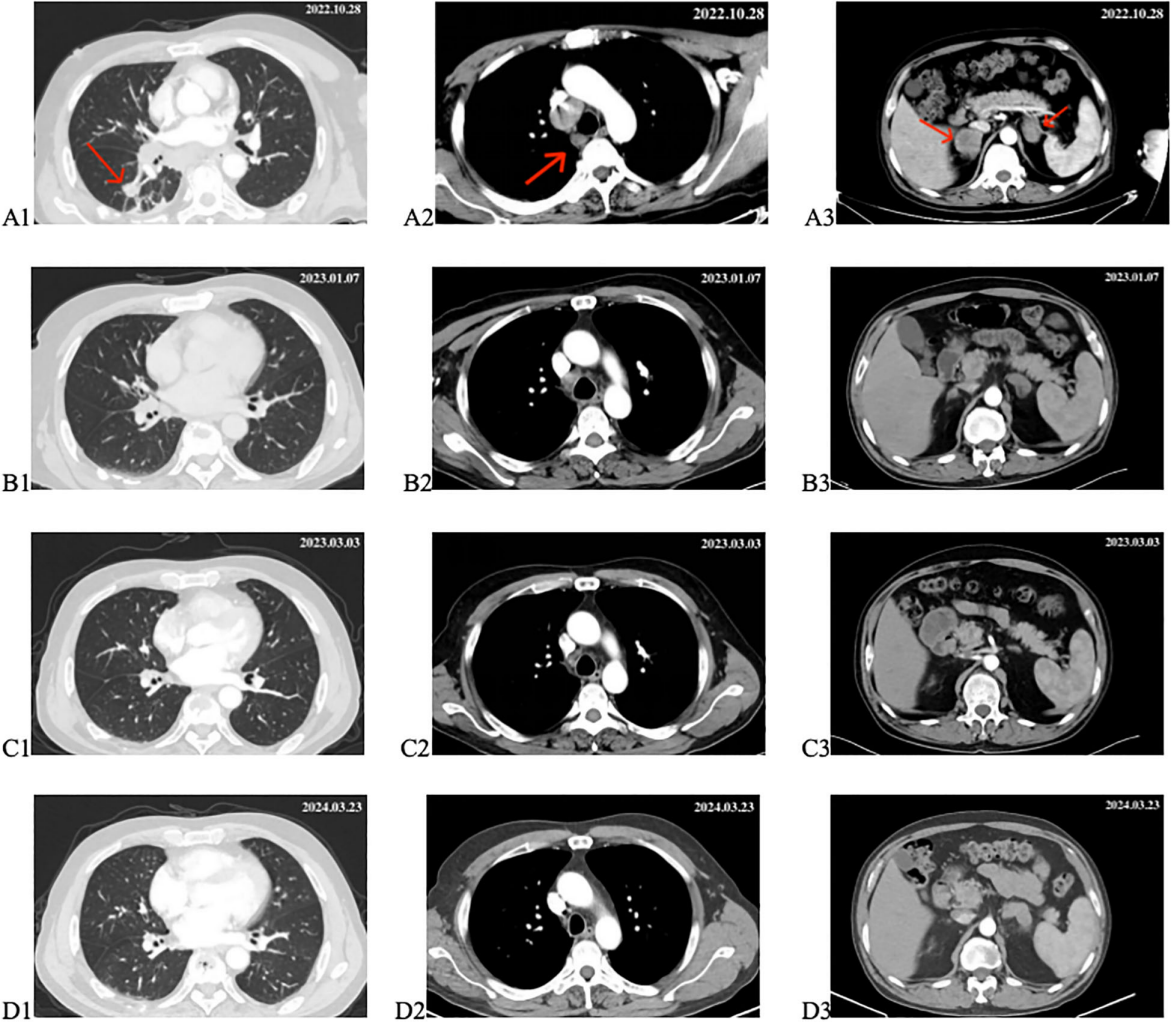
Changes in target lesion during treatment. (A1–A3) CT scan before treatment. (B1–B3) CT scan after 2 cycles of treatment. (C1–C3) CT scan after 4 cycles of treatment. (D1–D3) CT scan after 18 months of treatment. The red arrows mark the target lesions.
